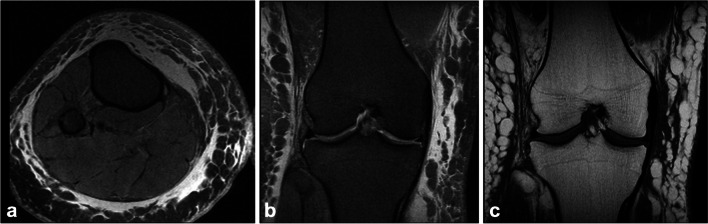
Forty-year-old woman with gradual onset of right knee pain and swelling. Axial (a) and coronal (b) PD FS and coronal T1 (c) MRI images demonstrate extensive diffuse subcutaneous edema with a clinical diagnosis of lymphedema since 13 years of age. Cellulitis and edema of other causes could have a similar appearance on MRI
